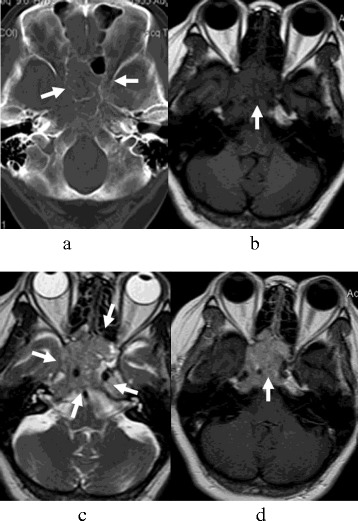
SNEC of paranasal sinuses in a 41-year-old man (a-d). The lesion was symmetrical, and the size was about 5.8 cm × 5.7 cm × 4.3 cm. (a) CT image showed worm-eaten bone destruction in sphenoid sinus, anterior cranial fossa, and orbital apex; however, bone contours still could be seen. (b) T_1_-weighted MR image demonstrated isointensity. (c) T_2_-weighted MR image demonstrated isointense together with a ‘pigeon’ pattern. (d) Contrast-enhanced T_1_-weighted MR image demonstrated a moderate heterogeneous enhancement mass, which showed involvement of the pharyngonasal cavity, orbital apex, pterygopalatine fossa, sella, cavernous sinus, internal carotid canal, and jugular foramen.
